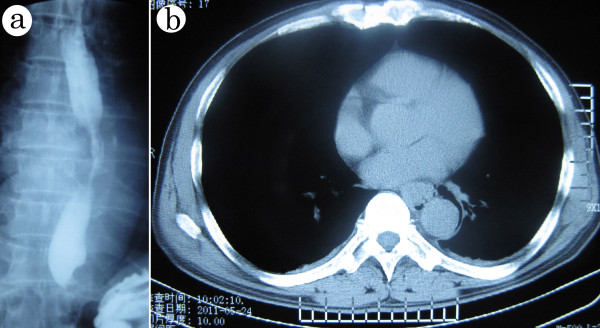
Imaging examinations of patients. (a) Irregular 6-cm narrowing of the middle esophagus in barium-swallow examination. (b). Thickened middle esophageal wall, with pneumomediastinum and bilateral effusion in CT scan. CT, computed tomography.
